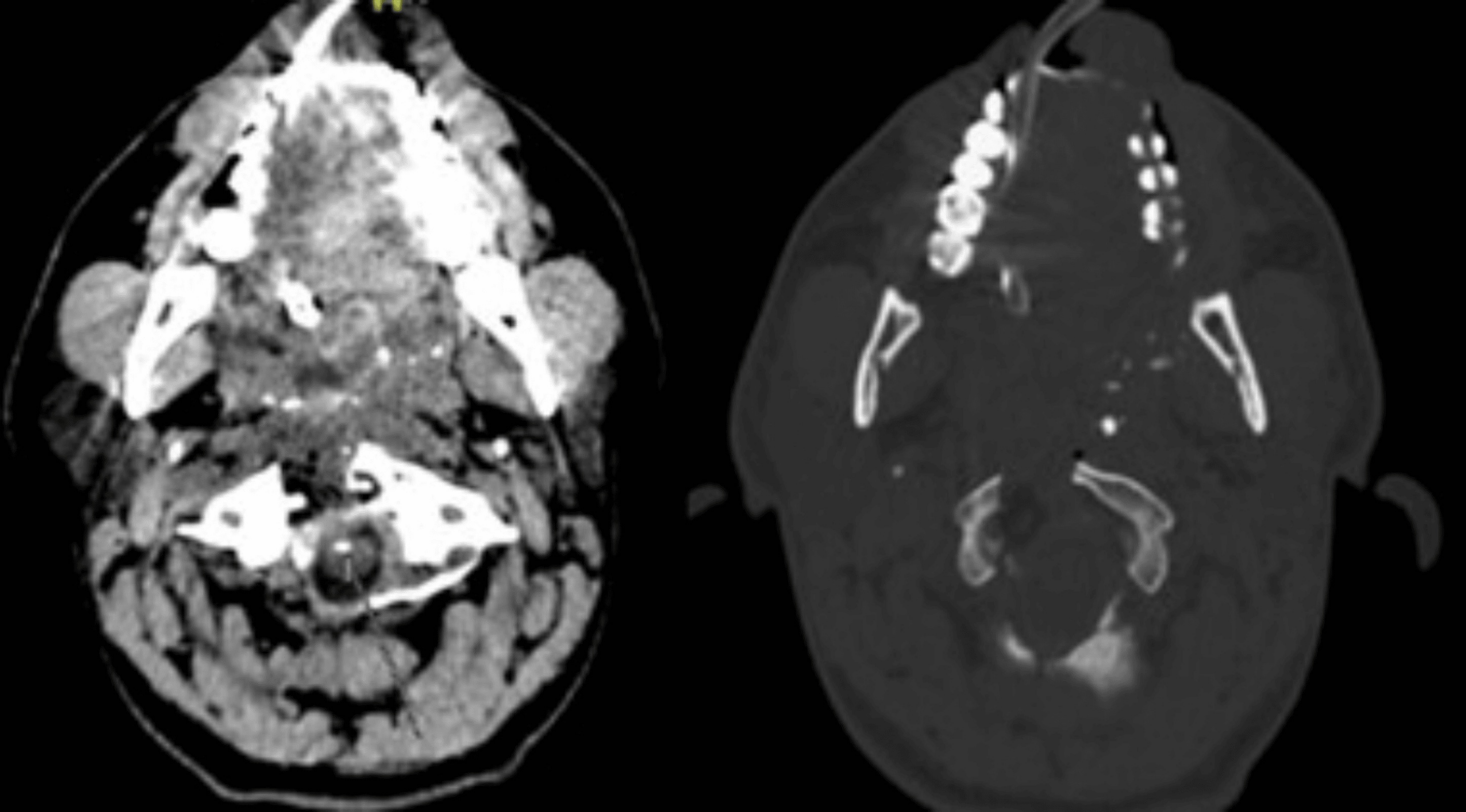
Postoperative axial CT scan demonstrating the successful removal of the hyperdense metallic foreign body (bullet) and decompression of the cervicomedullary junction. The anterior arch of C1 and the odontoid process of C2 show evidence of partial resection, with no residual bone fragments causing significant spinal canal narrowing. Soft tissue edema has resolved, and the surgical field appears clean, with no evidence of hematoma or infection. CT, computed tomography
